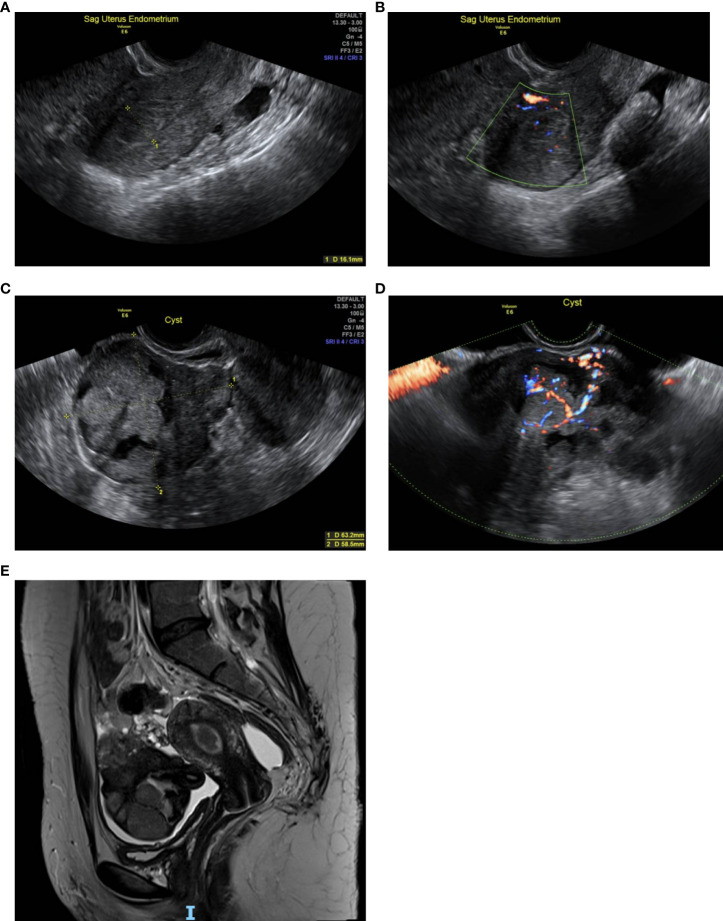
(A): Transvaginal scan (TVS) of the uterus shows an endometrial thickness of 16 mm. (B): TVS of the uterus shows anechoic areas with Doppler colour flow within the endometrial cavity. (C): TVS shows a right adnexal solid cystic mass measuring 74 x 58 x 63 mm, with the solid component measuring 40 mm. (D): TVS with colour Doppler, demonstrating moderate blood flow through the right adnexal mass. (E): Pelvic MRI shows a polypoidal lesion in the uterine cavity. The right adnexal mass is seen within a pool of free ascitic fluid extending both behind and in front of the uterus.
